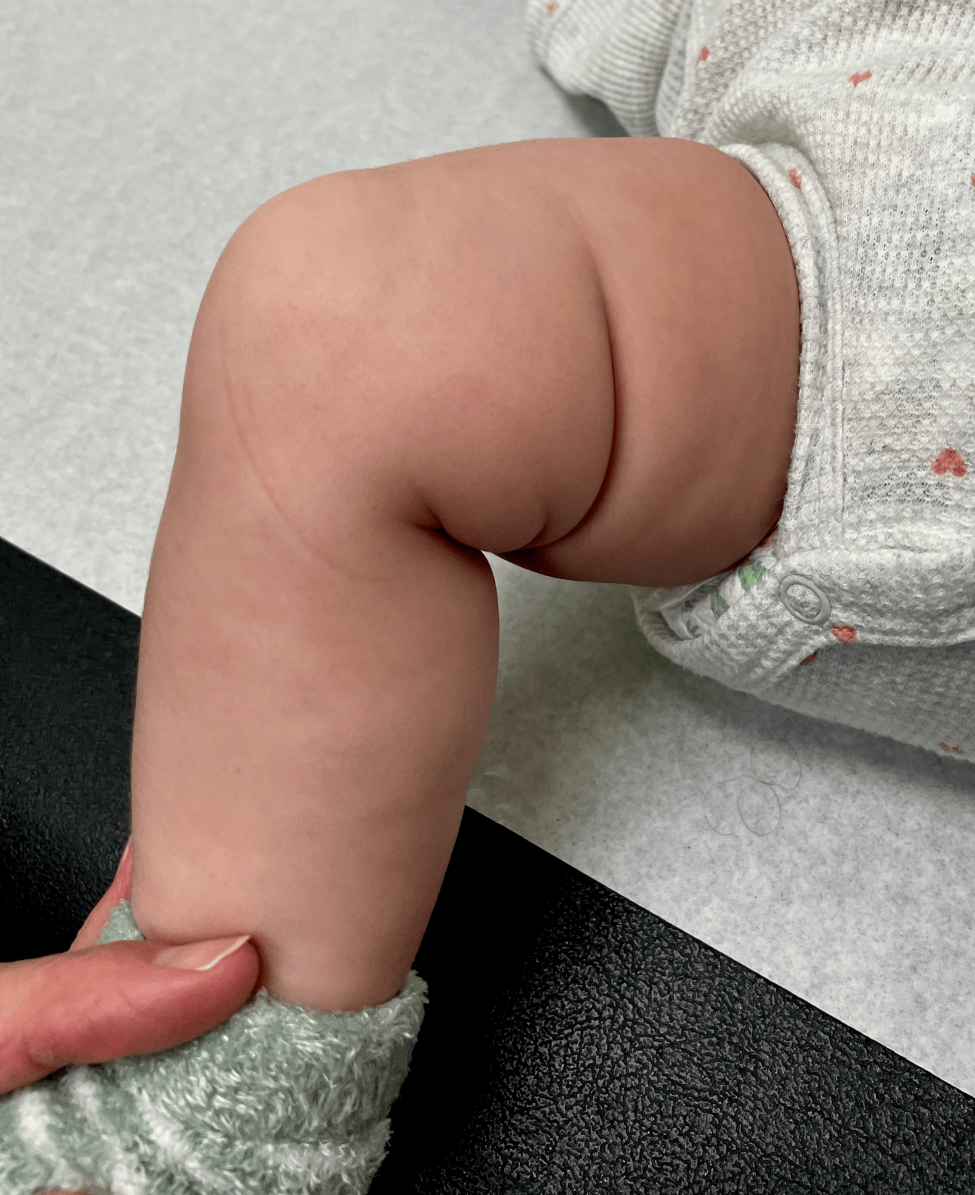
This 15-month-old girl was referred for a limp and a short limb This girl has an asymmetric medial thigh skin fold and a radiographically documented right hip dislocation. Image Credit: James G. Gamble
